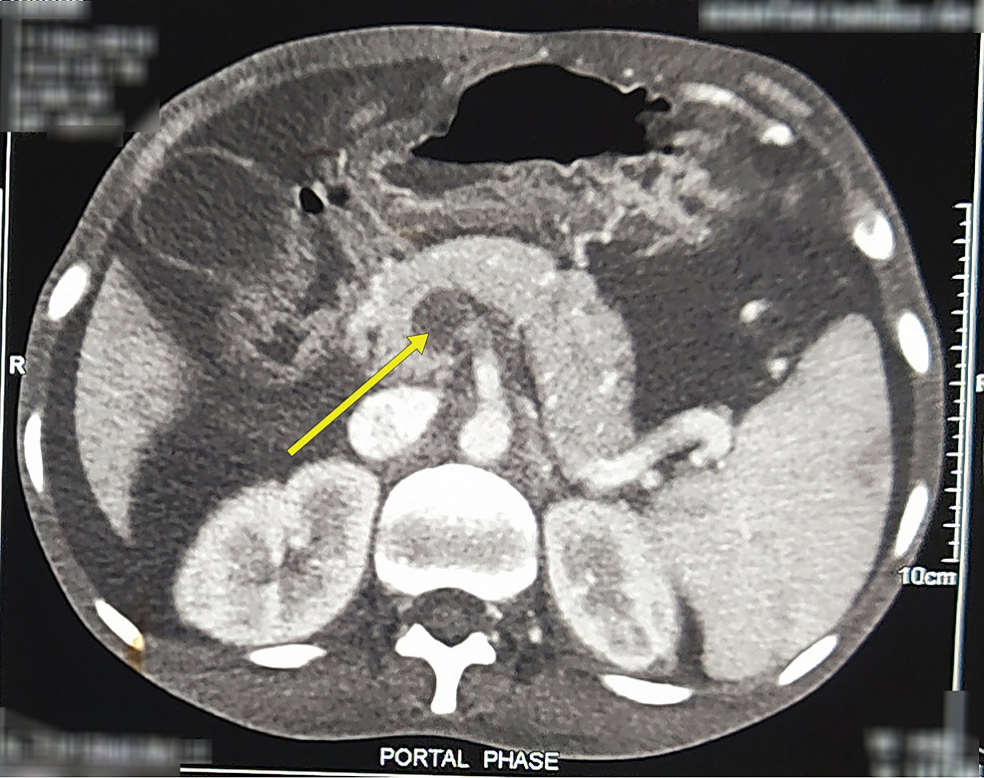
Axial view of contrast CT (portal phase) showing occluding thrombus in the splenic vein and the portal vein (yellow arrow). CT, computed tomography
